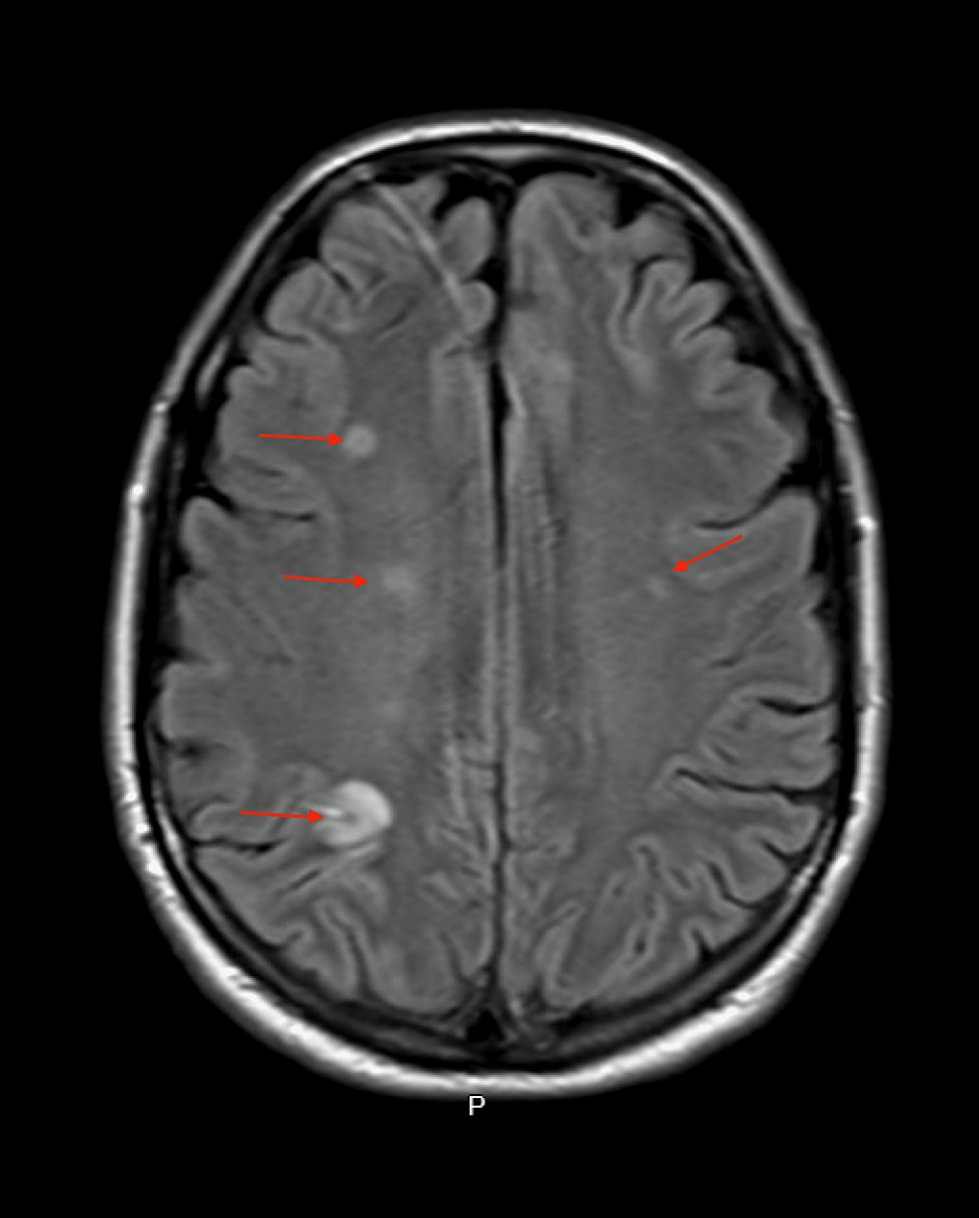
Magnetic resonance imaging of the brain showing multiple cerebral infarcts (red arrows)
